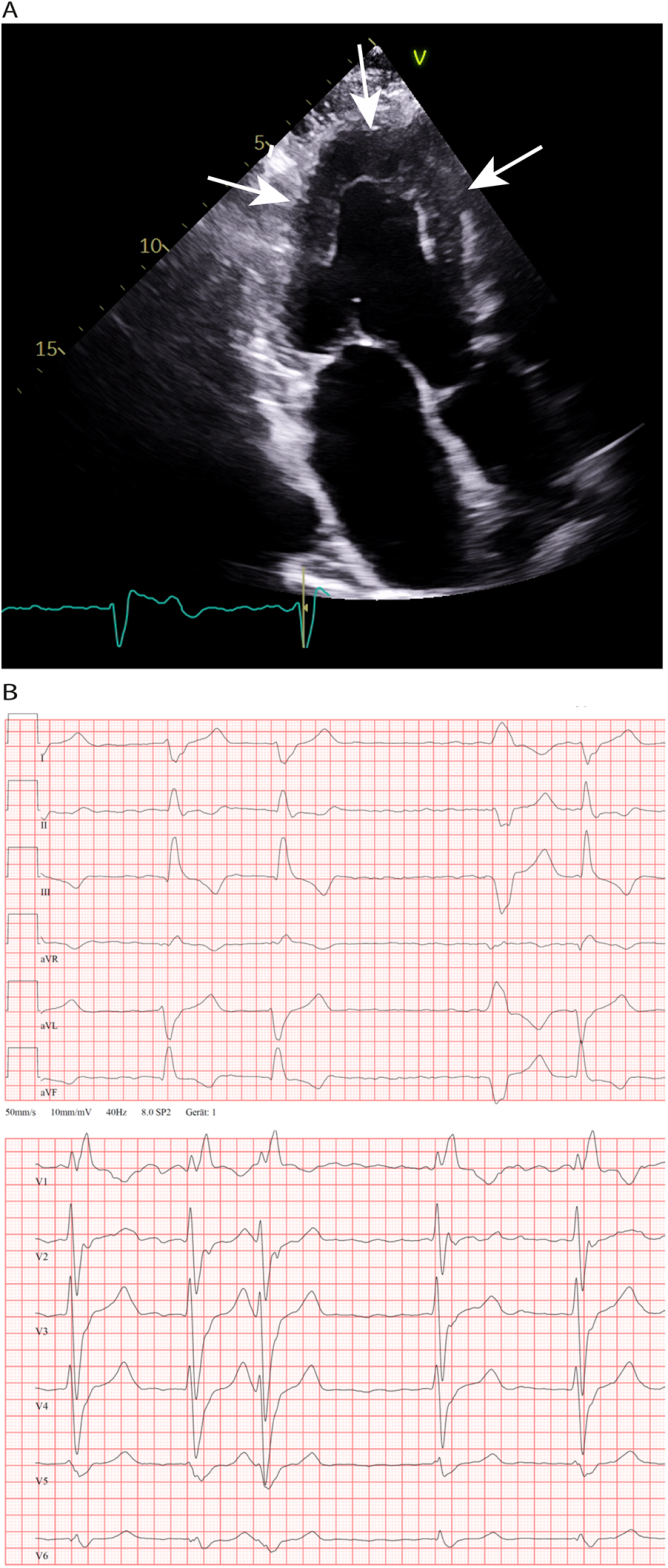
(A) Transthoracic echocardiography and 4-chamber view made in 2011; concentric LV hypertrophy (19 mm wall thickness) an LA enlargement. (B) Chest leads during an episode of atrial fibrillation at the age 60 years. Right bundle branch block, in addition marked right axis deviation (not shown) and absence of low voltage QRS complexes.
